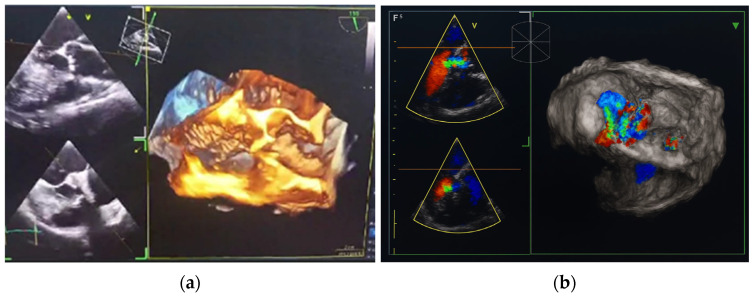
Transesophageal heart ultrasound 3D imaging. (a) 3D reconstruction of the aortic root and ascending aorta. (b) 3D Color Doppler imaging of aortic regurgitation.
